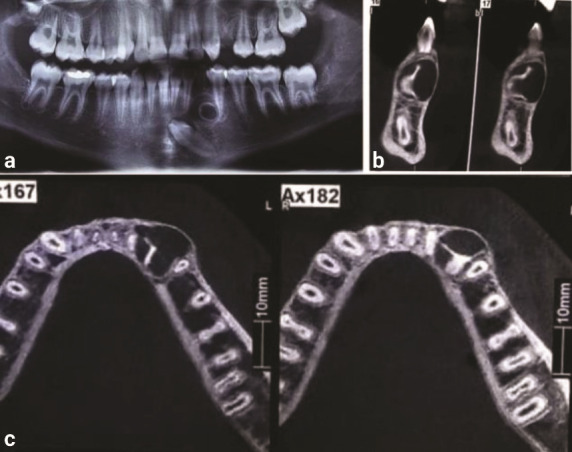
a: Panoramic radiography shows a ring-shaped (doughnut-like) radiopacity, associated with a radiolucent
